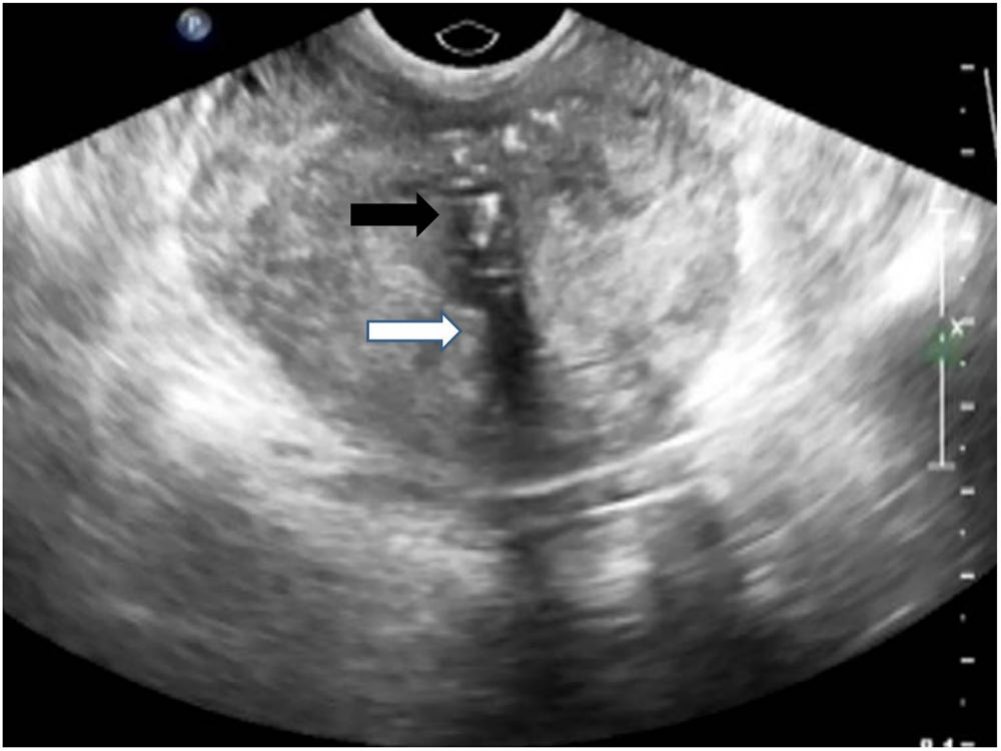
Prostatic calcifications with acoustic shadowing. Legend: The ultrasound image of patients with prostate calcification on the first clinic visit The prostate calcification appeared as the hyper echogeneous foci (black arrow). The acoustic shadowing appeared as the dark tail (white arrow)
